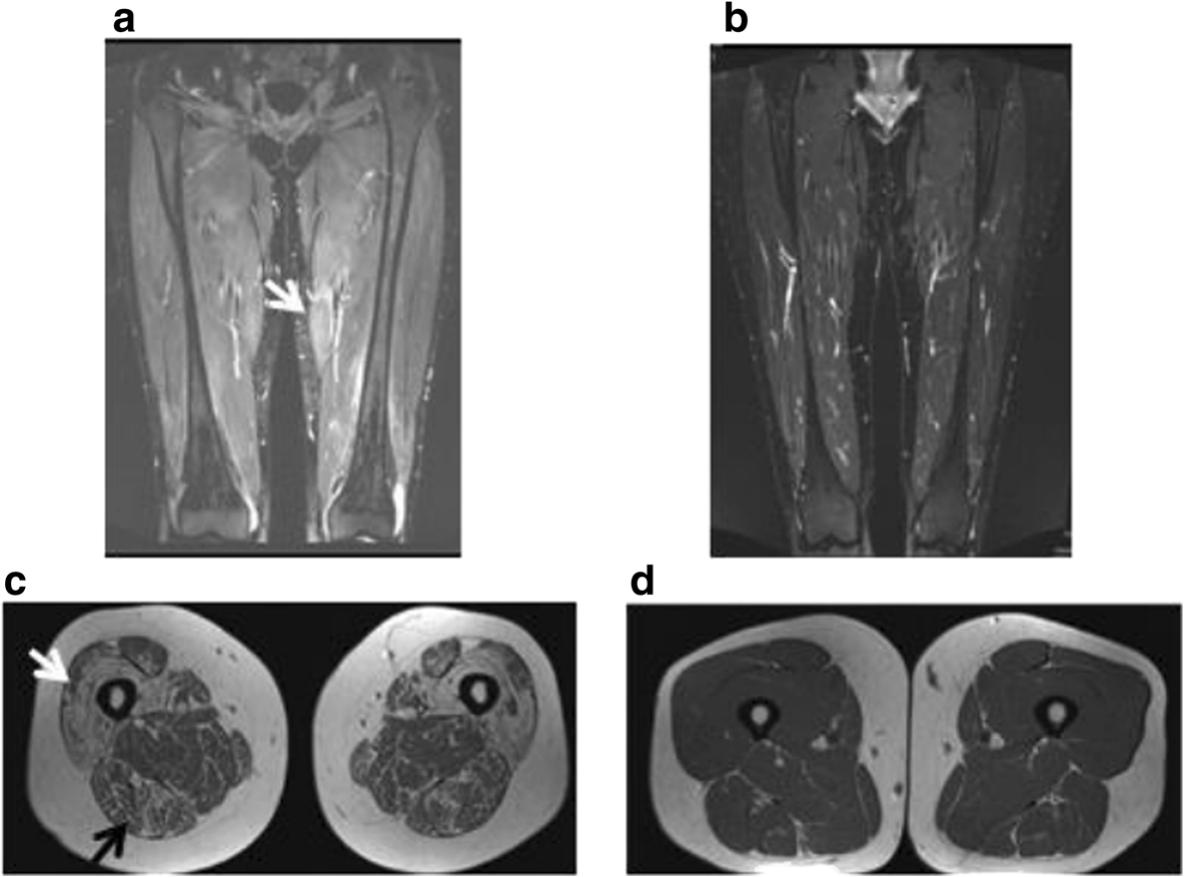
a Muscle MRI with coronary STIR sequence of the thighs in a patient with ASS. Compared with surrounding tissue, the muscles are generally brighter in color, most evident in the medial compartment (*arrow*), indicating general edema. The patient was scored with 36/36 points in the muscle edema score. b The correspondent control to the patient in (a) with less color difference between muscles and surrounding tissue indicating no muscle edema (score 0). c Axial T1-weighted image in an ASS patient with almost total fatty replacement anteriorly (Gouttalier score 4, *light arrow*) and fatty streaks with more muscle than fat tissue (Gouttalier score 2, *dark arrow*) posteriorly. d The correspondent control to the patient in (c) evaluated as normal muscle tissue (Goutallier score 0–1)
